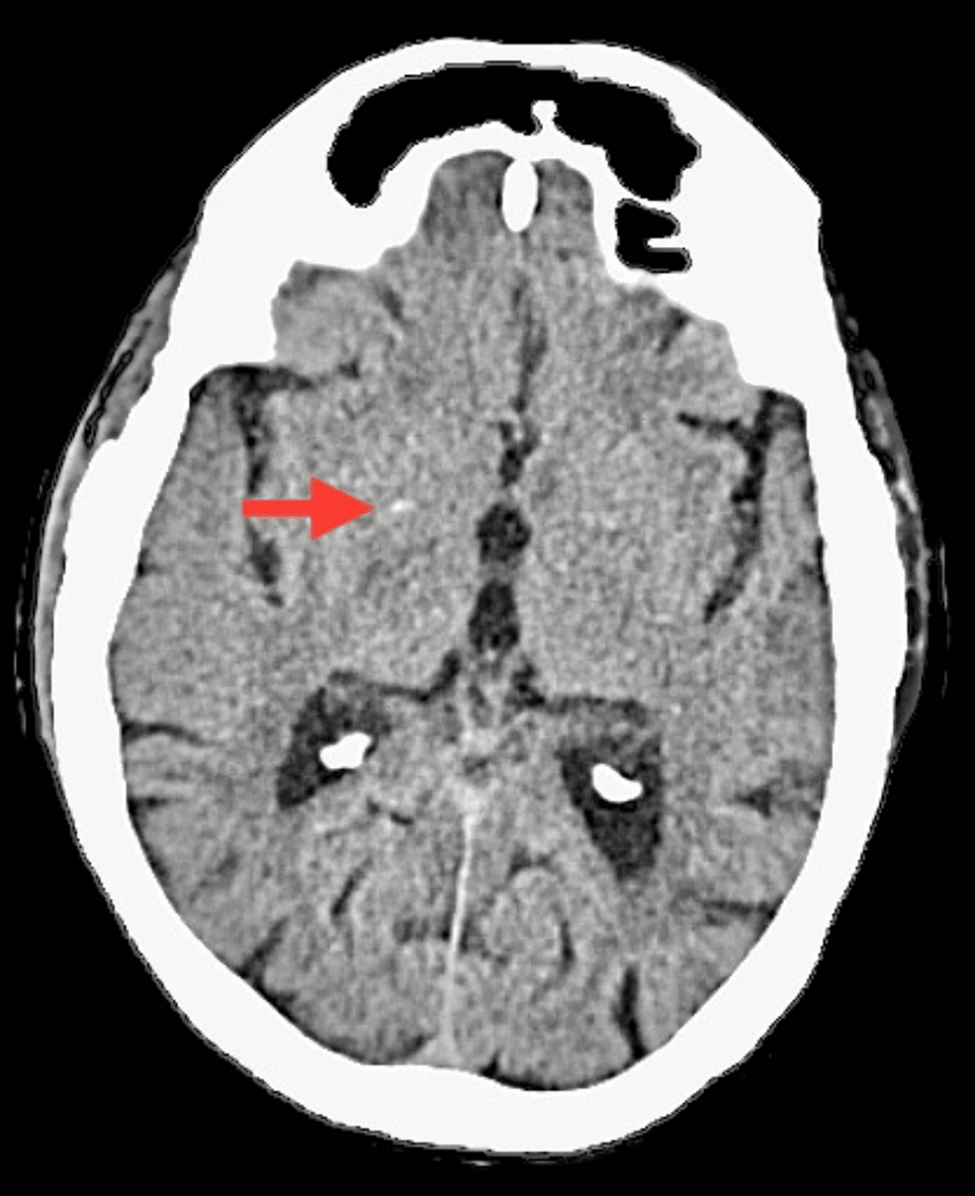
CT scan of the brain The red arrow shows an infarct in the right corona radiata.
